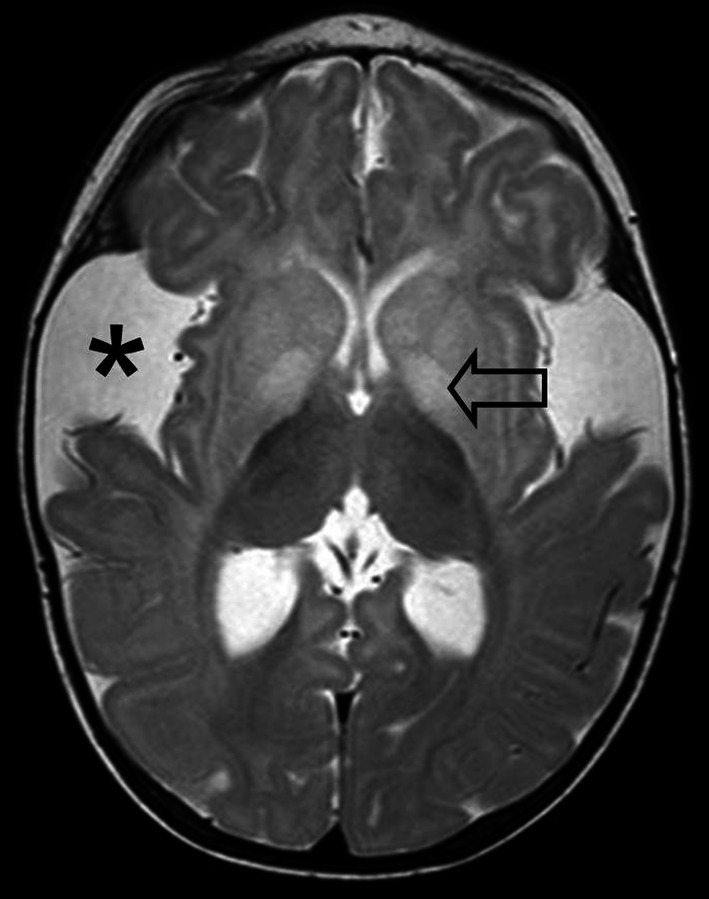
Six month old girl with known GA1. T2 weighted MRI (TR 3944, TE 80, Flip angle 90°, slice thickness 3 mm) shows widening of the Sylvian fissures (*asterisk*) and a high signal intensity of the basal ganglia (*arrow*)
